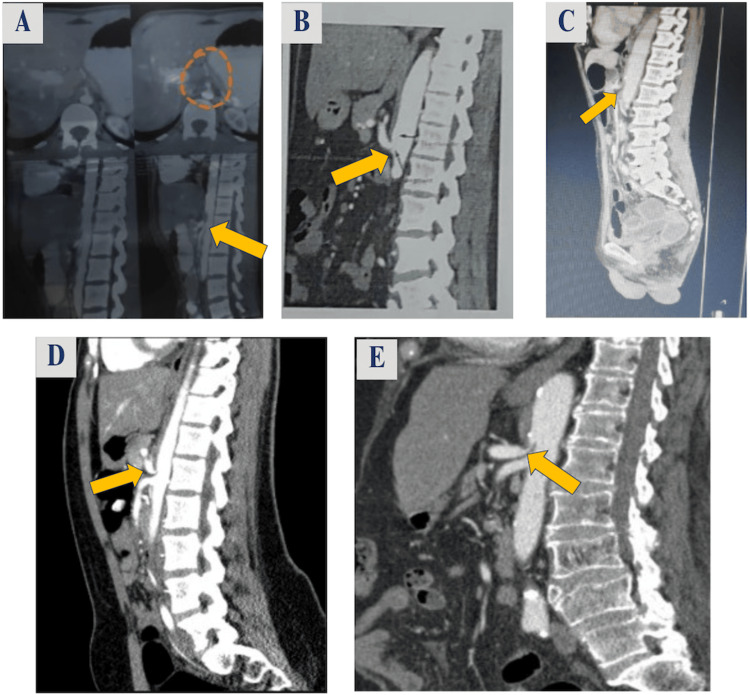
Pre-operative CECT abdomen images. The figure depicts pre-operative CECT images of five patients (A)-(E) showing stenosis of the celiac artery with post-stenotic dilatation (indicated by the yellow arrows). CECT: contrast-enhanced computed tomography.
